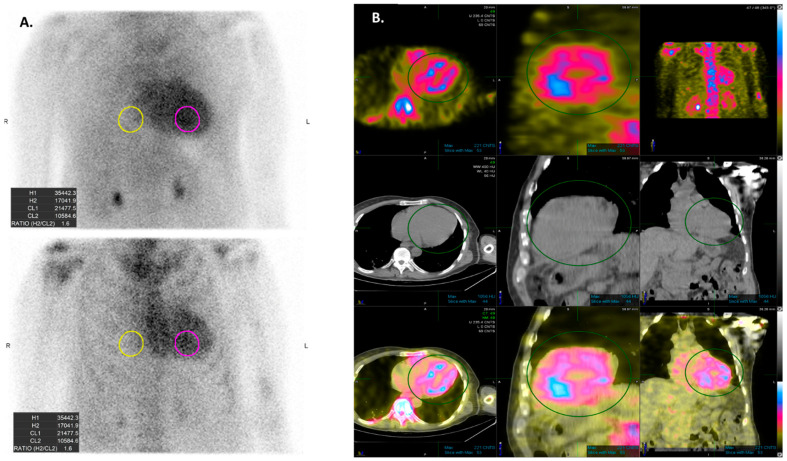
Technetium-labeled cardiac scintigraphy (PYP scan) is commonly used for the diagnosis of cardiac amyloidosis, especially for transthyretin cardiac amyloidosis. (A) depicts planar imaging with the use of a semiquantitative evaluation using the heart to contralateral ratio. (B) shows SPECT imaging, which allows visualization of the difference in the radiotracer uptake between the left-ventricular blood and the myocardium.
